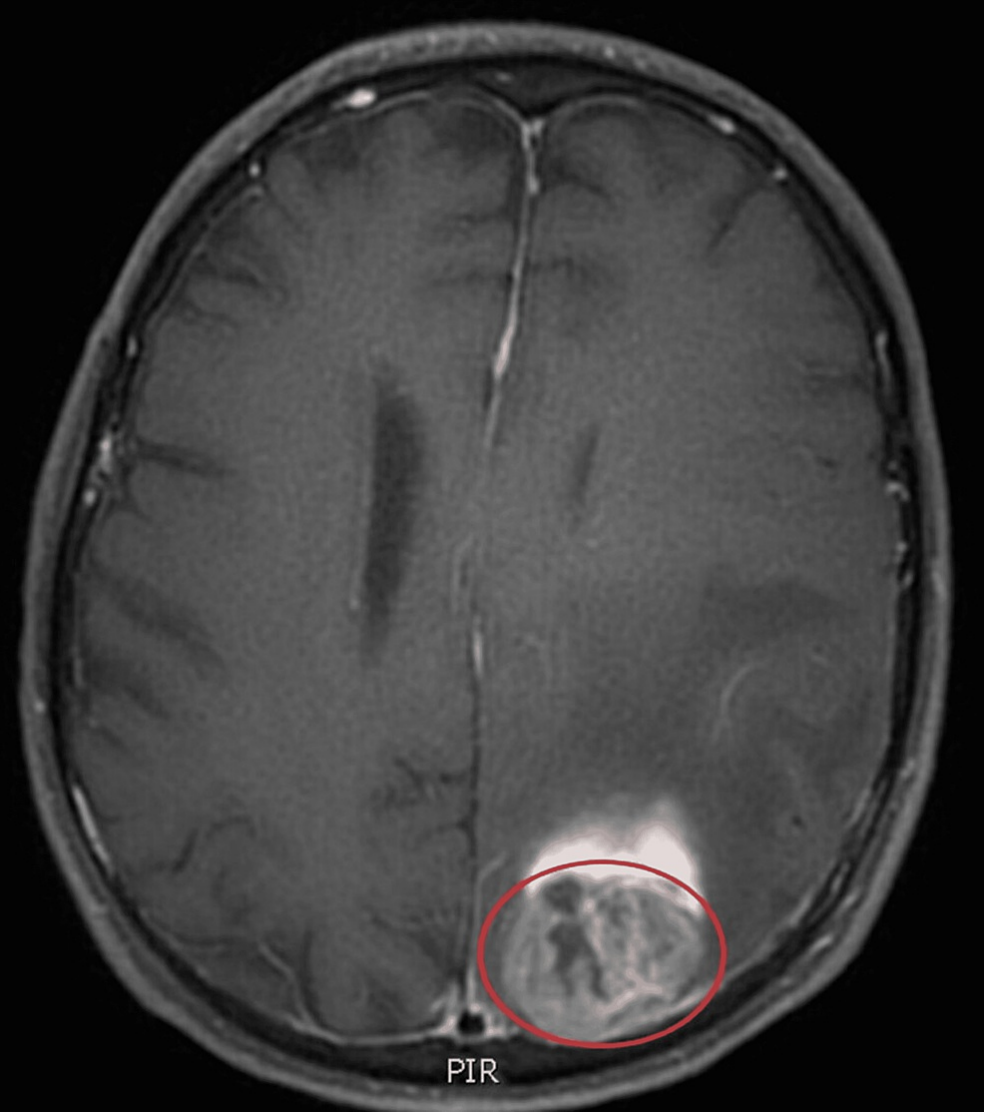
MRI Brain with contrast enhancement T1 image showing central clearing, heterogeneous contrast enhancement with rim enhancement. Consistent with glioblastoma multiforme.
